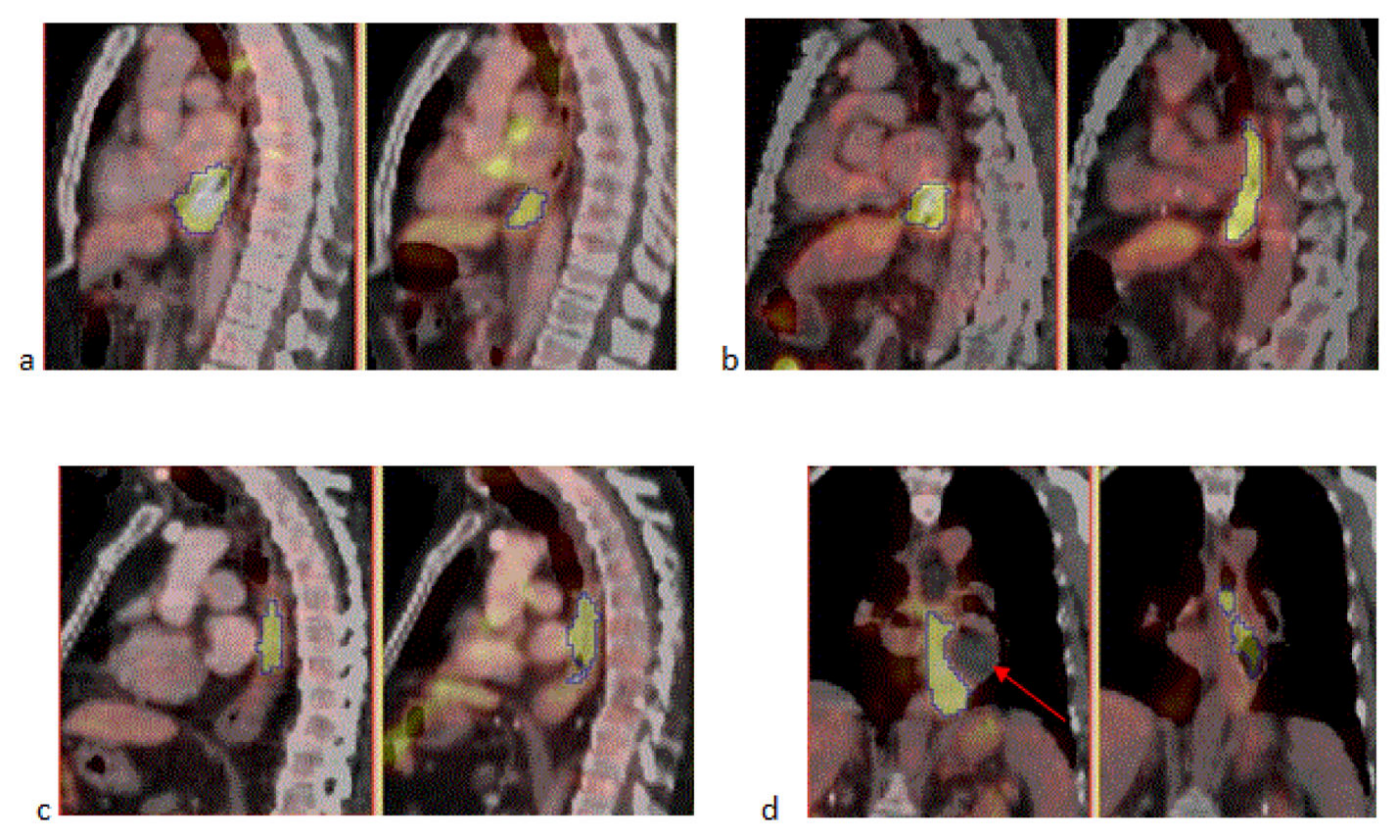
FDG PET/CT images of four patients pre-CRT (left) and post-CRT (right). The contours indicate the original MAVs on pre-CRT images and residual MAVs on post-CRT images, respectively. (a) Patient 6, BinA=100%, overlap=33%, (b) Patient 4, BinA=43%, overlap=44%, (c) Patient 3, BinA=37%, overlap=53%, (d) Patient 2, BinA=14%, overlap=5%.
